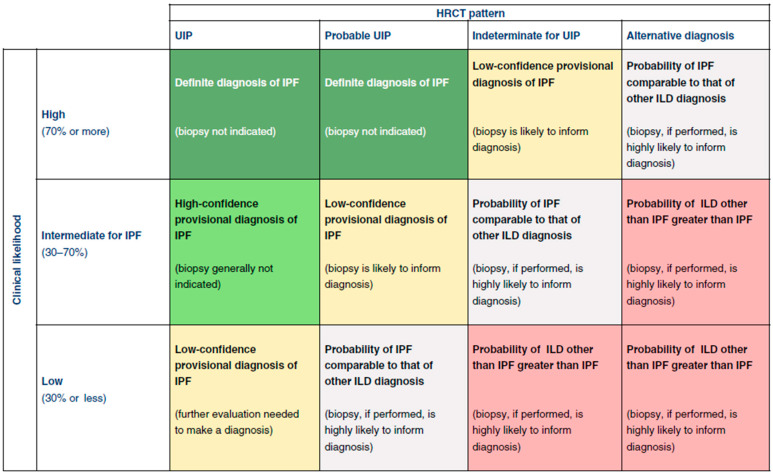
Probability of non-invasive diagnosis of IPF based on clinical diagnosis and radiographic data [11]. Legend. HRCT, high-resolution computed tomography; UIP, usual interstitial pneumonia; IPF, idiopathic pulmonary fibrosis. Reprinted with permission of the American Thoracic Society. Copyright C 2025 American Thoracic Society. All rights reserved.
